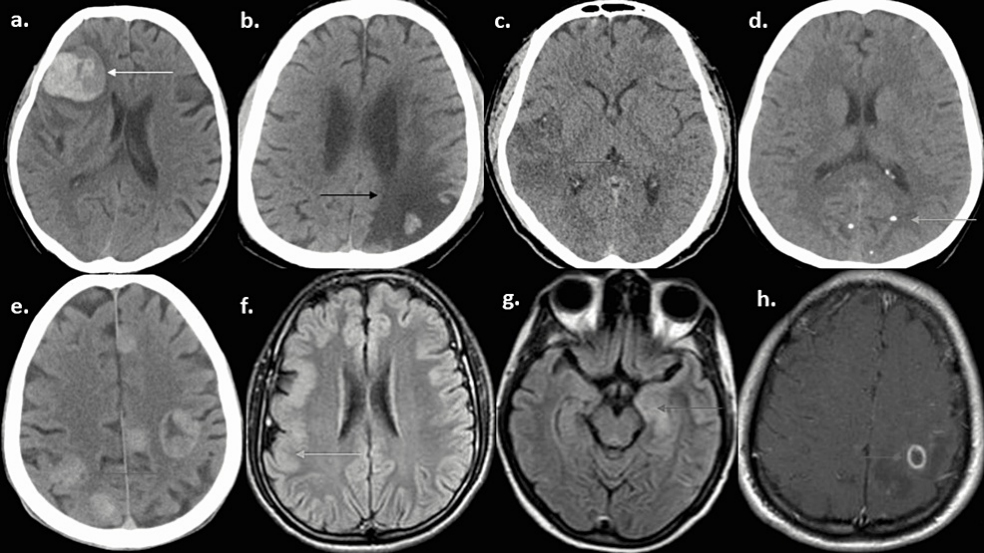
Computed tomography brain axial sequences showing (a) intracranial hemorrhage (white arrow), (b) chronic infarct in left parietal-occipital region (black arrow), (c) acute infarct in right parietal region (red arrow), (d) calcified stage of neurocysticercosis (orange arrow), (e) multiple brain metastases (green arrow). MRI T2 FLAIR axial sequences showing (f) focal cortical dysplasia in right hemisphere (yellow arrow), (g) bilateral mesial temporal hyperintensities in herpes encephalitis (purple arrow). MRI T1 post-contrast sequence showing (h) ring-enhancing lesion of tuberculoma (blue arrow). MRI: magnetic resonance imaging, FLAIR: fluid-attenuated inversion recovery.
